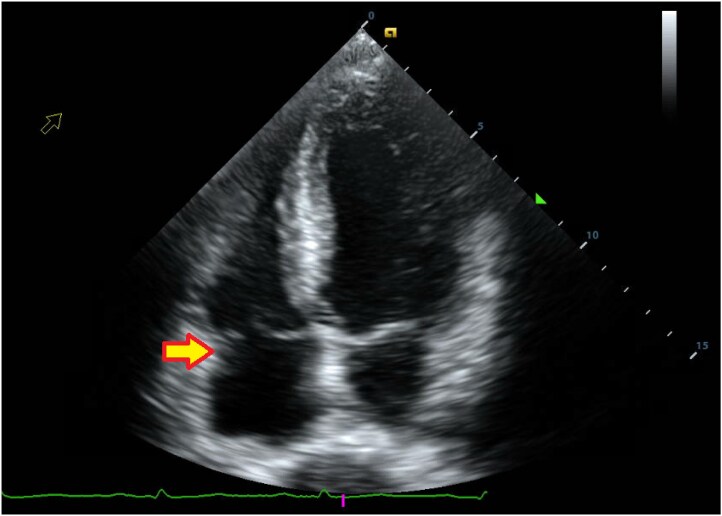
Post-procedural apical four-chamber TTE showing RA after extraction of vegetations and masses. Very small residual vegetation along the RA (arrow).
